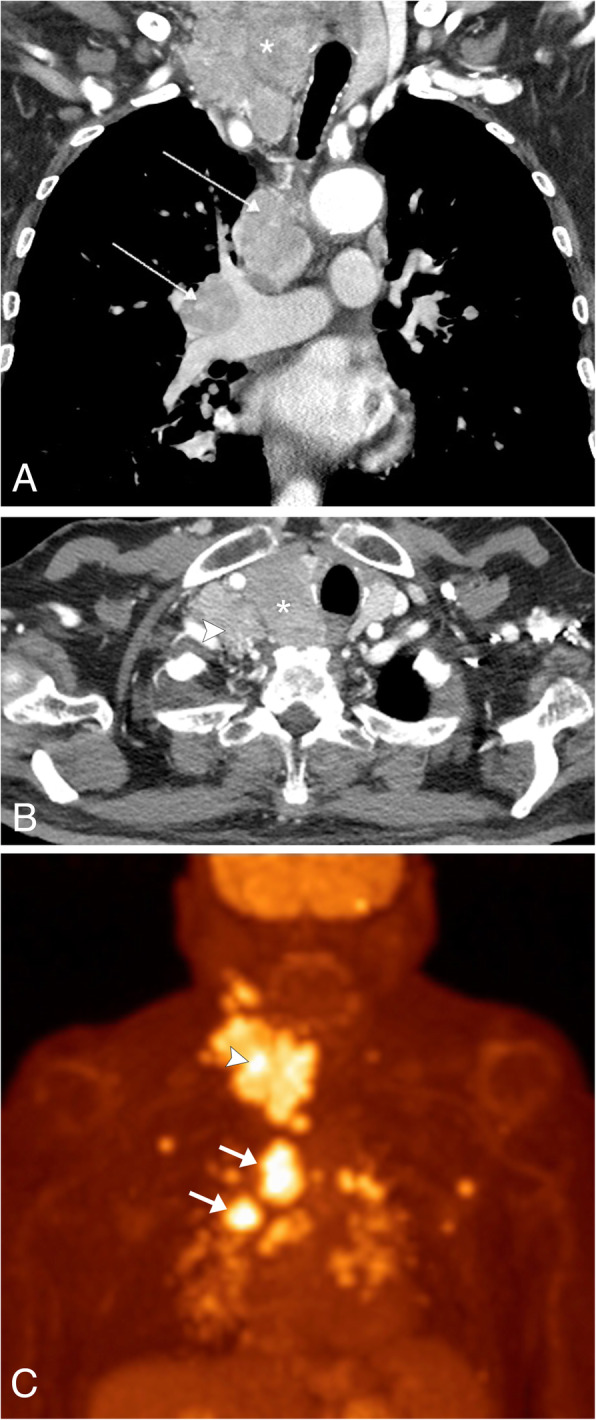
Thyroid CA. Contrast-enhanced CT coronal (a) and axial (b) images demonstrate a large mass in the right lower neck associated with an enlarged and heterogeneous right thyroid lobe (asterisk), which is causing leftward shift of the trachea. Enlarged right neck base, mediastinal and right hilar lymph nodes (arrows) are noted. Biopsy of these lesions confirmed the presence of metastatic thyroid cancer. c Coronal FDG-PET/CT shows avid hypermetabolic uptake in the right neck base mass (arrowhead) and within the metastatic lymph nodes within the neck and chest (arrows)
